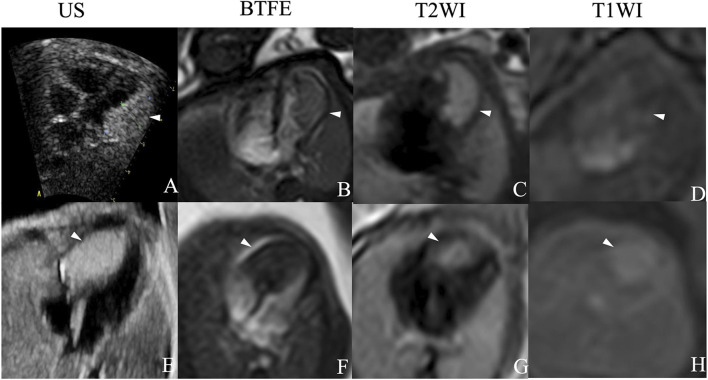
Fetal cardiac rhabdomyoma: Ultrasound and MRI findings. Cardiac rhabdomyomas appear as hyperintense signals on T2WI against the dark background of the myocardium, a phenomenon often referred to as the "bright spot" sign. The white arrow represents the lesion. (A–D) Rhabdomyoma in the left ventricular wall; (E–H) rhabdomyoma in the interventricular septum.
